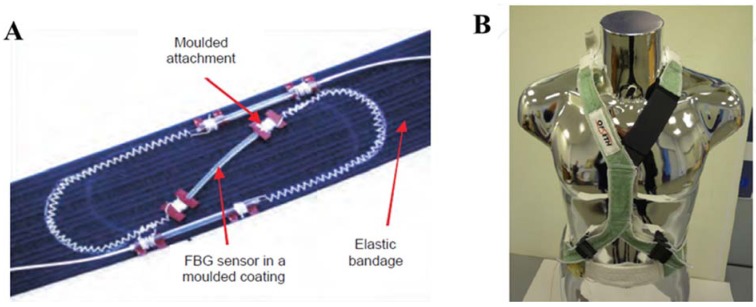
(A) Design of the FBG sensor developed in OFSETH project (adapted from [57]); (B) MRI-compatible sensing harness which embeds the fiber optic sensors for respiratory monitoring (adapted from [57]).
